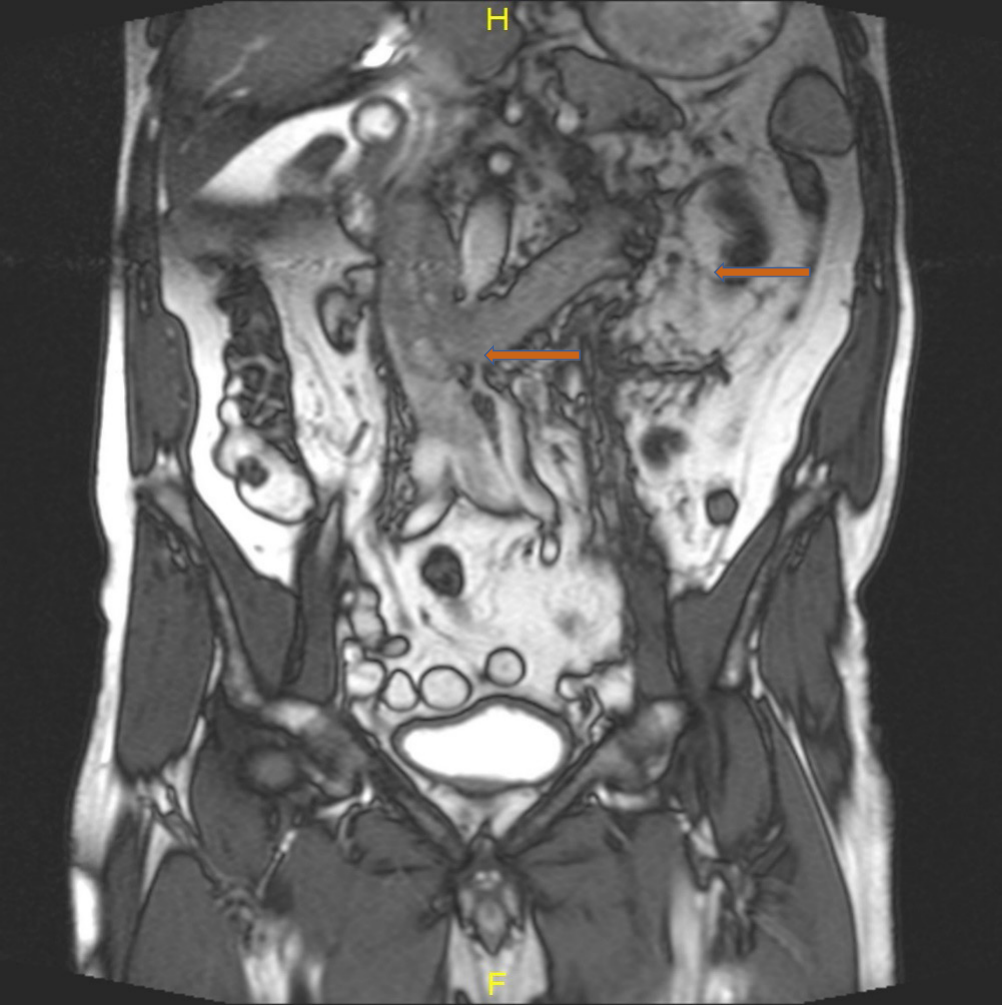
MRI of the abdomen and pelvis showing left renal mass, renal vein, and IVC thrombus.
